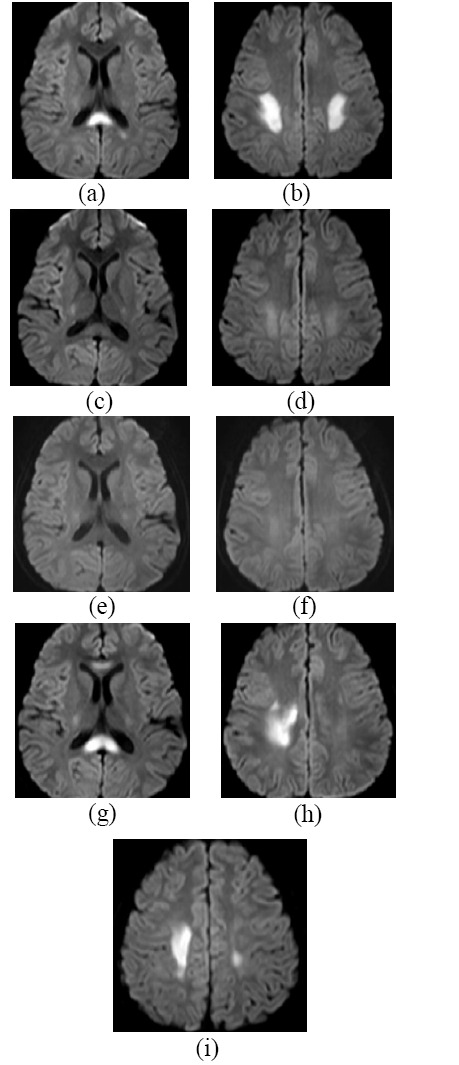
Male patient with cytotoxic lesions of the corpus callosum. a, b. Diffusion weighted imaging (DWI) on March 26^th^, 2014, showing abnormal high signal in the splenium of the corpus callosum and the deep white matter at the junction of the bilateral fronto-parietal lobes; c, d. On April 5^th^, 2014, the abnormal high signal on DWI was significantly reduced; e, f. Follow-up DWI on June 27^th^, 2015, appeared normal; g-i. DWI on March 13^th^, 2016, revealed an abnormally high signal in the splenium and genu of the corpus callosum, as well as the deep white matter at the junction of the bilateral fronto-parietal lobes (more prominent on the right side).
